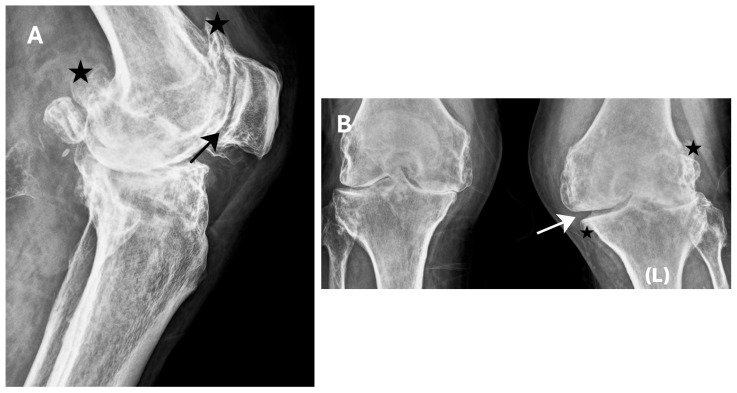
X-rays of the knee joints. (A) Lateral X-ray of the left knee joint. (B) Standing anteroposterior X-ray of both knee joints. Advanced degenerative changes. Significant valgus deformity. The joint space (white arrow) is significantly narrowed and asymmetric between the medial and lateral sides. Numerous osteophytes (black asterisks). The patellofemoral joint (black arrow) is significantly narrowed.
